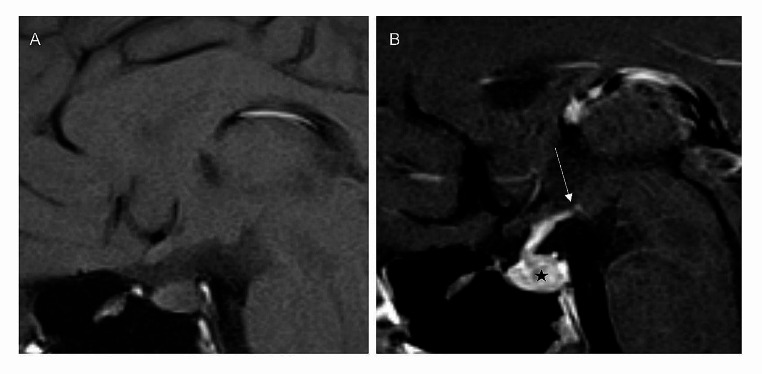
The first MRI of a patient with diabetes insipidus. (A) Sagittal non-contrast T1-weighted image shows the absence of the posterior pituitary bright spot. (B) Sagittal contrast-enhanced T1-weighted image with 2 mm slice thickness shows a small germinoma (black star) in the posterior pituitary lobe and inferior pituitary stalk, with a subtle thickening of the floor of the third ventricle. Please note that the contrast enhancement extends to the mammillary bodies (arrow), indicating a pathological condition. The diagnosis was confirmed by surgery 19 months later
